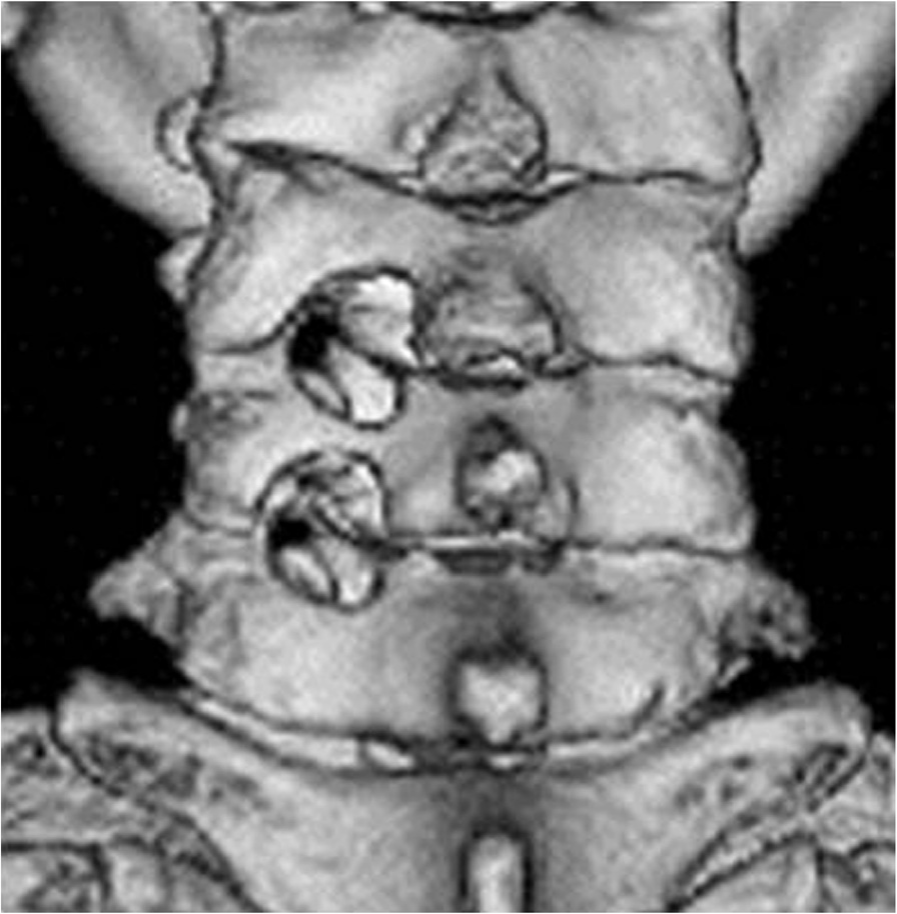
Postoperative 3D CT view. Tandem keyhole foraminotomy was performed in C5/6/7. Half of the facet joints were preserved. The lateral border of vertebral body was recognized through the decompressed hole.
